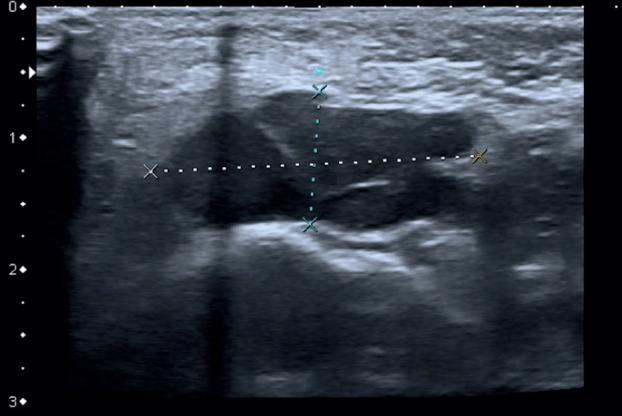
Ultrasound images. Lesion on B mode. Echography of the left cheek: polilobulated hypoechogenic lesion, well defined and embedded in deep layer of subcutaneous tissue anterior to masticatory muscles without infiltration of approximately 1x2x3 cm. It is compressible. On its lateral left side, there are two small vessels with phasic flow suggestive of veins and it does not present flow on Doppler Mode. Taking into account the clinical and radiologic correlate, the lesion is suggestive of a thrombosed venous malformation.
